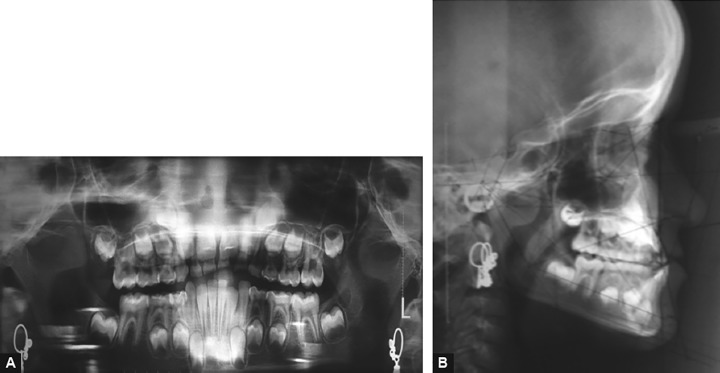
Pretreatment radiographic images: Orthopantomogram (OPG) and cephalogram
